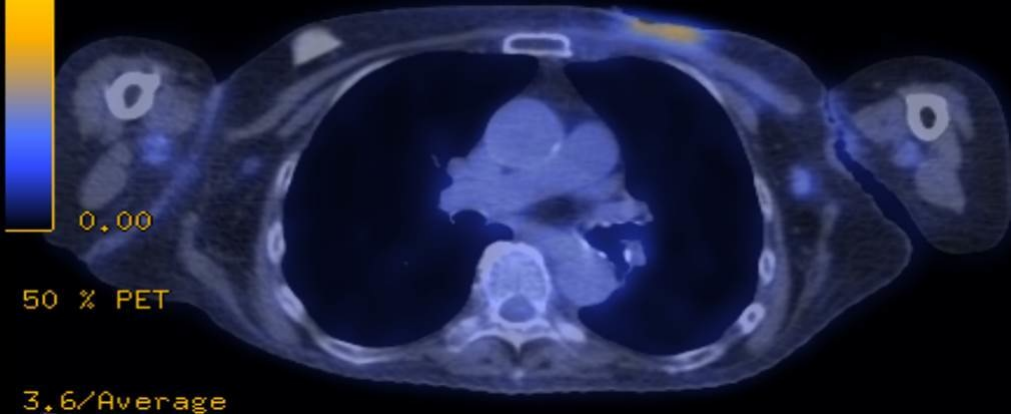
Cross-sectional positron emission tomography/computed tomography (PET/CT) imaging at 18 months post chest wall radiation showing ongoing local control of the chest wall disease.
